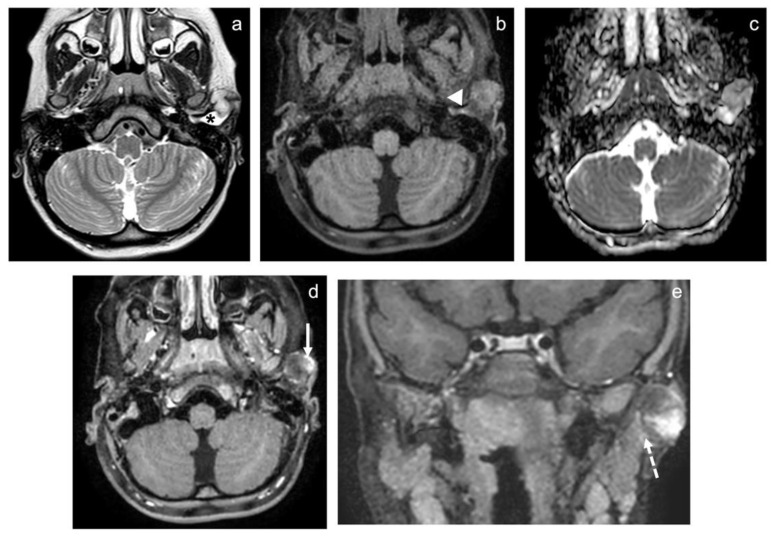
(a–d) Axial MRI FLAIR and traditional sequences. * and the white arrows show the mass and its relationship with surrounding tissue. (e) Coronal view shows (white interrupted arrows) the relationship of the mass with the parotid fascia and the gland.
